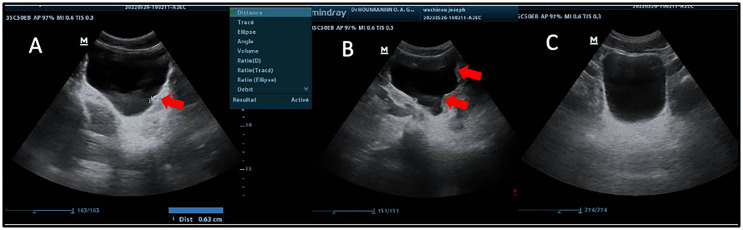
Ultrasound images showing some lesions observed in the bladder of patients with (*Sh*+) or without (*Sh*−) *S. haematobium* infection. A: Bladder of irregular shape and thickened wall (red arrow); B: Bladder of irregular shape with pseudopolyps (red arrow); C: Normal bladder; M: Male patient.
